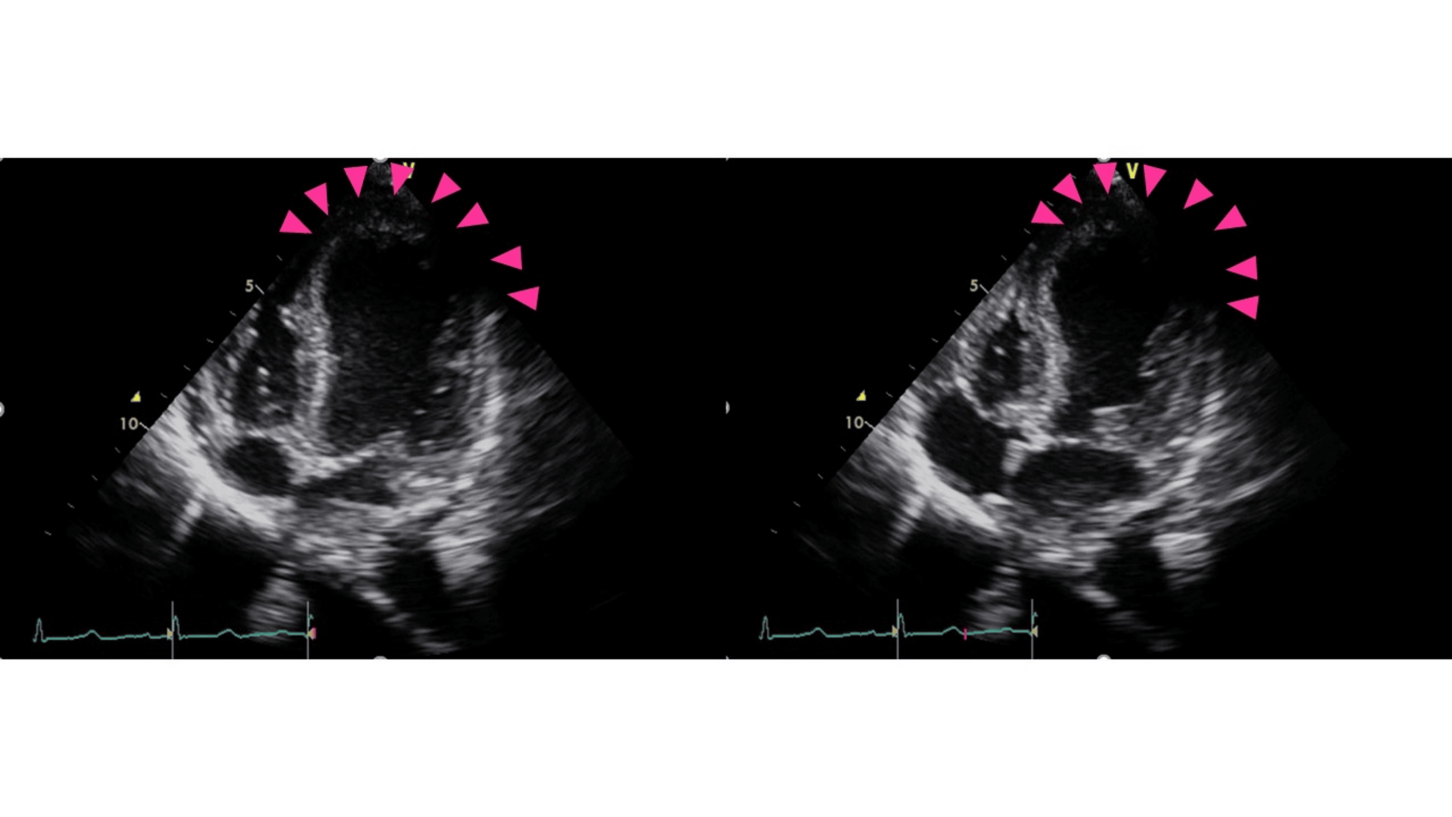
Transthoracic echocardiography at admission Left panel: Diastolic phase; Right panel: Systolic phase
